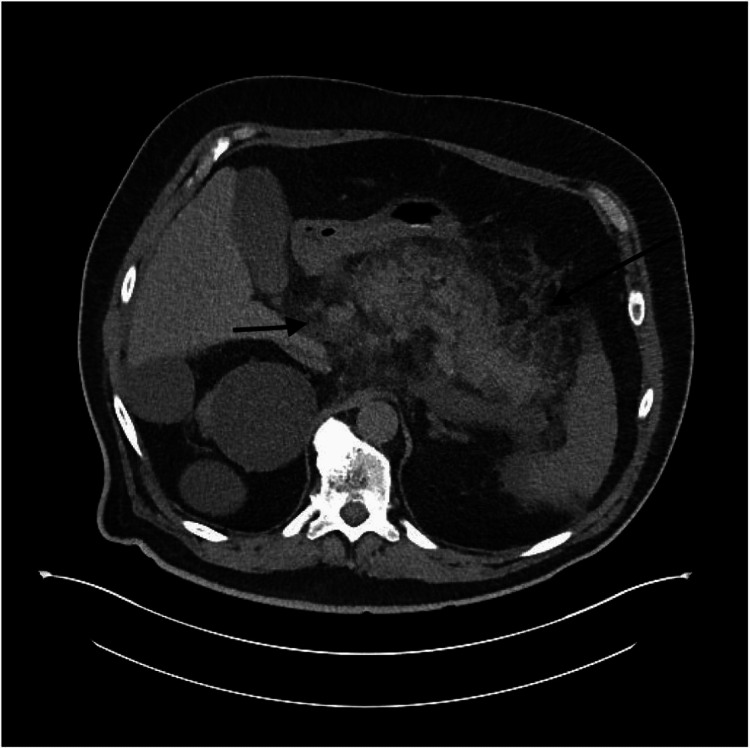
CT abdomen and pelvis showing inflammation in the whole pancreas
